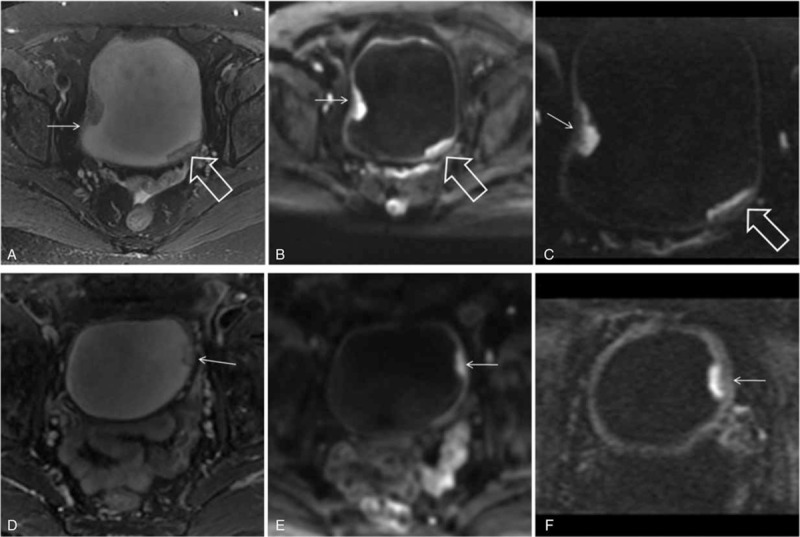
A 51-year-old man with multiple stages of pT1 urothelial carcinoma (A–C) and a 70-year-old man with stages of pT1 papillary urothelial carcinoma (D–F). A, D, The axial T2-weighted MRI shows elongated oval masses along the right bladder wall (arrow) and the left lateral wall (arrow and open arrow). The SI of the muscle layer at the base of the 3 tumors is slightly elevated, and the low SI line was disrupted. B, E, The axial fFOV DW MRI shows 2 high SI tumors without a submucosal stalk, but with a smooth tumor margin and submucosal thickening (arrow and open arrow). C, F, The axial rFOV DW MRI shows the 2 high SI tumors with a thickened submucosa (arrow and open arrow). DW = diffusion-weighted, fFOV = full field of view, MRI = magnetic resonance imaging, rFOV = reduced field of view.
